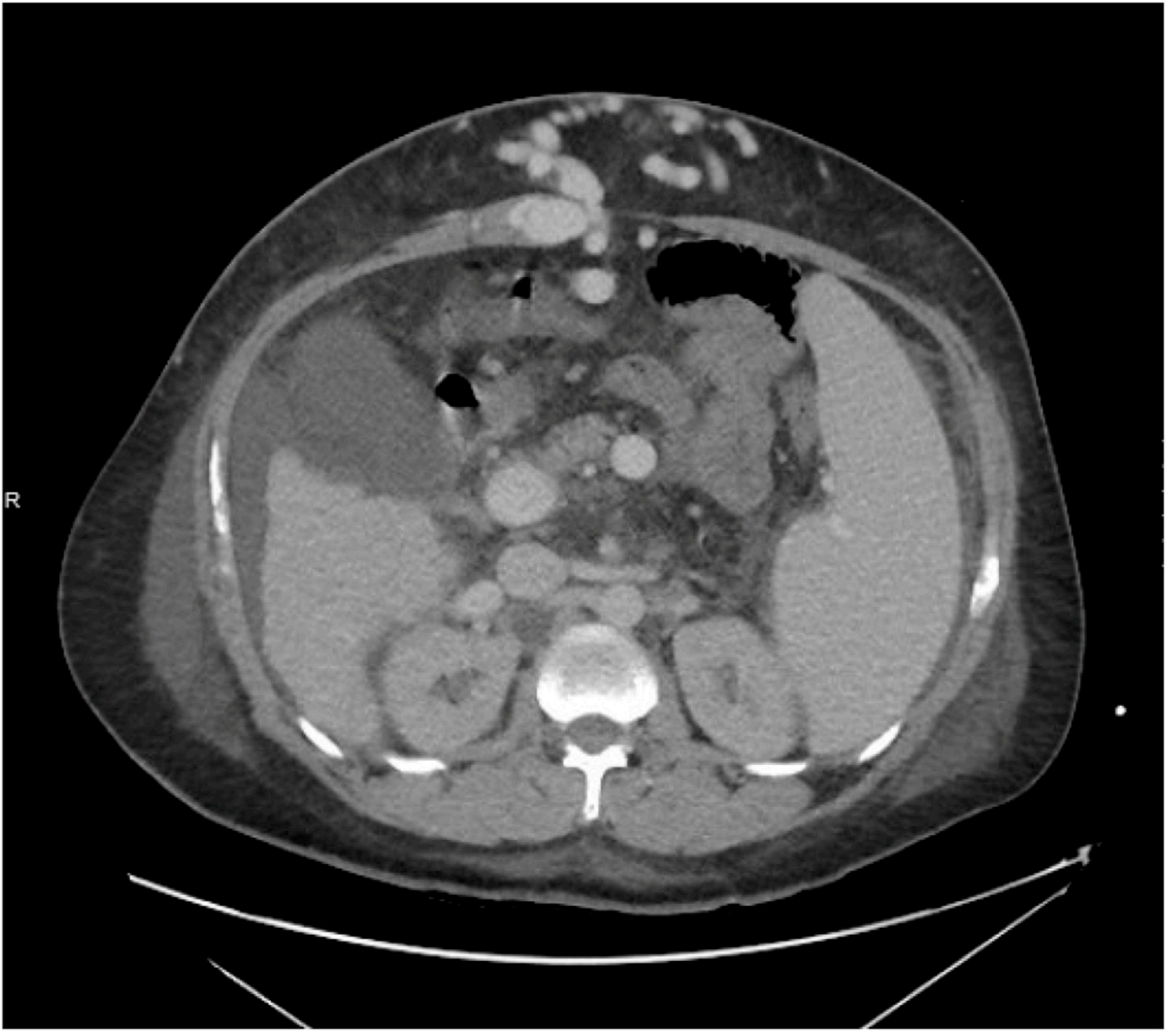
Example of a CT scan showing varices within the hernia sac and splenomegaly (contraindications to robotic hernia repair).
